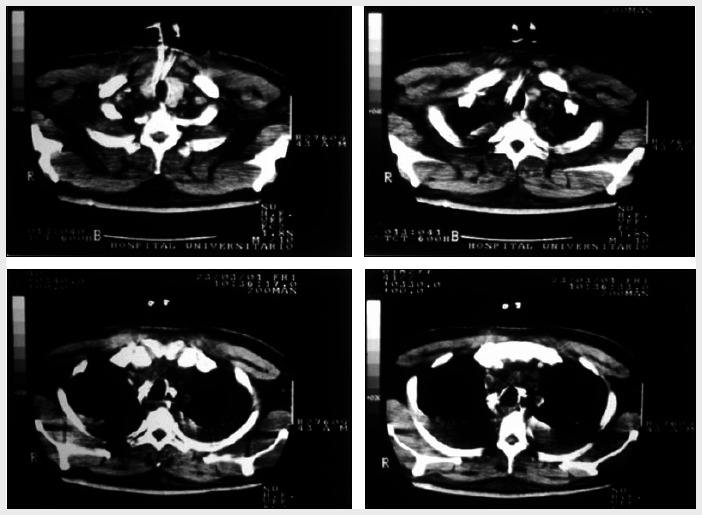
Chest computed tomography scan demonstrating mediastinal collection.
